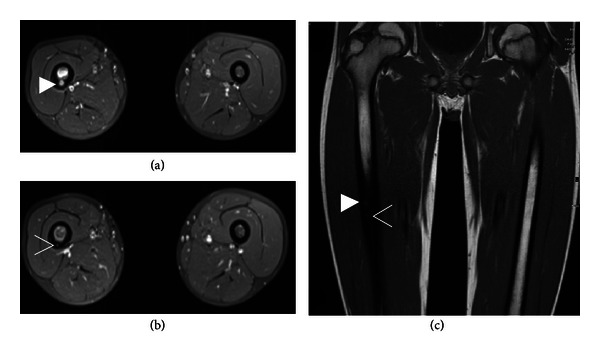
Contrast-enhanced MRI reveals a multicentric osteoid osteoma with two nidi in the femur diaphysis with a larger nidus of 6 × 5 mm (solid arrowhead in (a) and (c)) and a smaller 2 × 2 mm nidus inferiorly (open arrowhead in (b) and (c)). Note the thickened cortical bone and the bone marrow edema of the right femoral diaphysis. (a) and (b) contrast-enhanced T1 fs MRI; (c) T1 TSE MRI.
